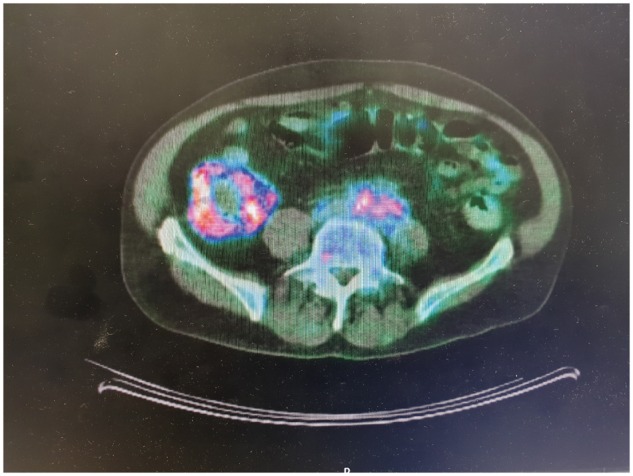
PET-CT scan of the same patient as in Fig. 1, demonstrating metabolic activity of the soft tissue
